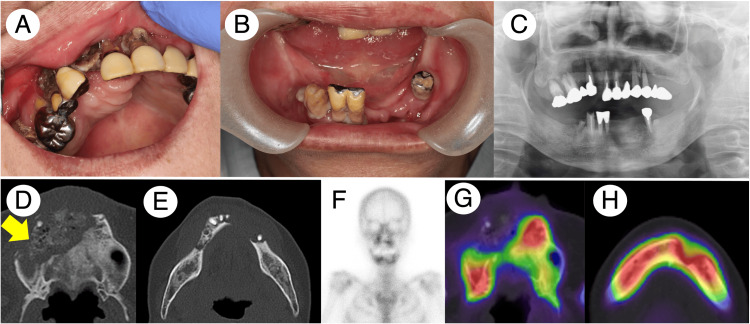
Patient 2 clinical and radiographic findings before the treatment. Intraoral examination found extensive bone exposure in both maxillary and mandibular bones (A and B). Panoramic X-ray imaging exhibited an irregular margin of the alveolar bone in both the maxillary and mandibular bones (C). MDCT revealed the extensive formation of the sequestrum and apparent sclerotic changes in the affected areas (the yellow arrow indicates the sequestrum in the maxillary bone) (D and E). Bone scintigraphy and SPECT showed the increased accumulation of 99mTc-MDP/HMDP in the affected areas (F, G, and H). MDCT, multidetector computed tomography; SPECT, single-photon emission computed tomography; 99mTc, technetium-99m; MDP, methylene diphosphonate; HMDP, hydroxy-methylene diphosphonate
